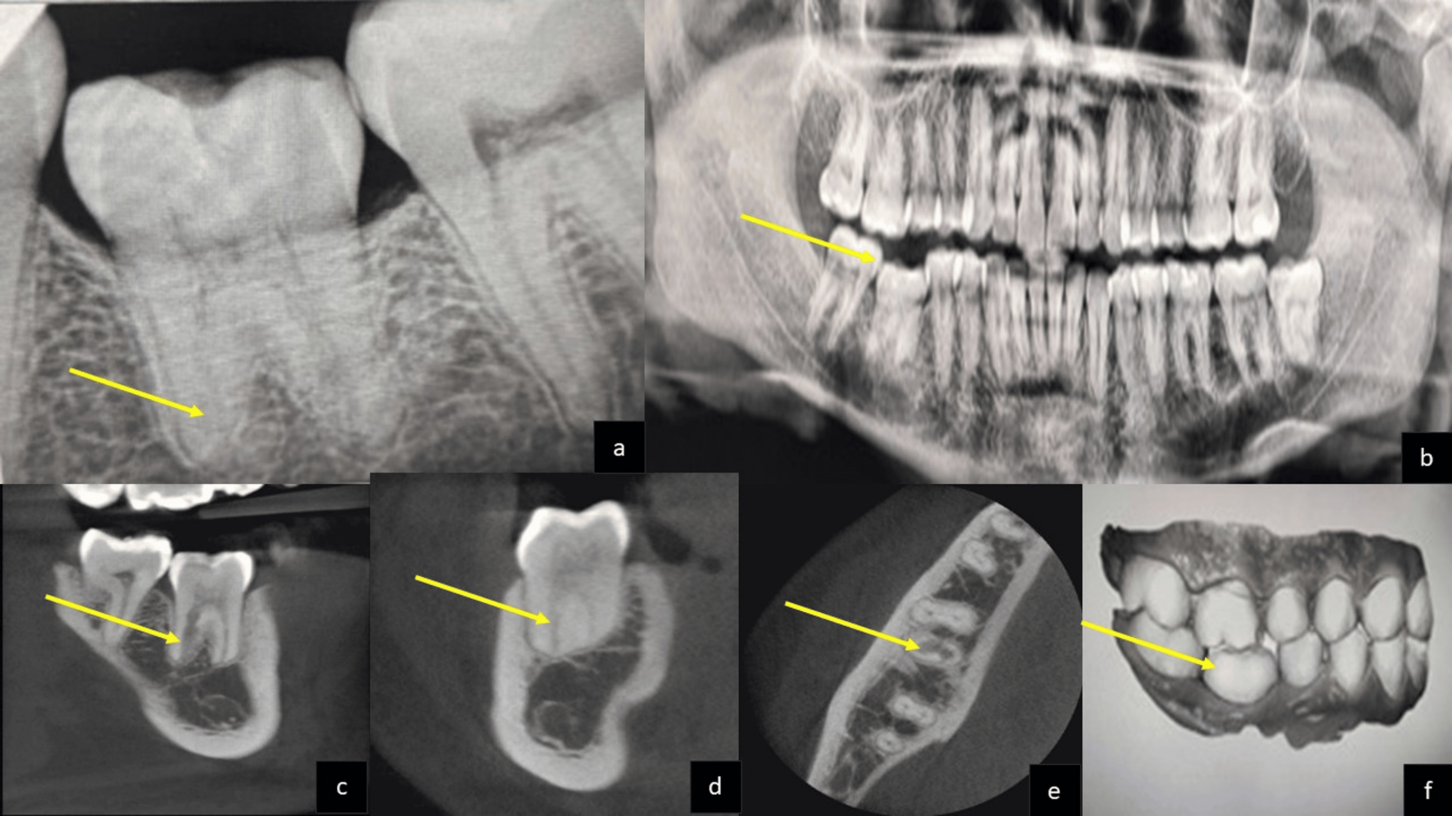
(a) Pre-operative RVG shows indistinct distal root outline and absence of lamina dura, (b) pre-operative OPG-submerged 46, 16 supraerupted, (c) pre-operative CBCT sagittal view, (d) coronal view, (e) axial view, and (f) pre-operative intraoral scanning image-approximately 0.5 mm space can be appreciated between the two molars. RVG: radiovisiography; OPG: orthopantogram; CBCT: cone beam computed tomography.
